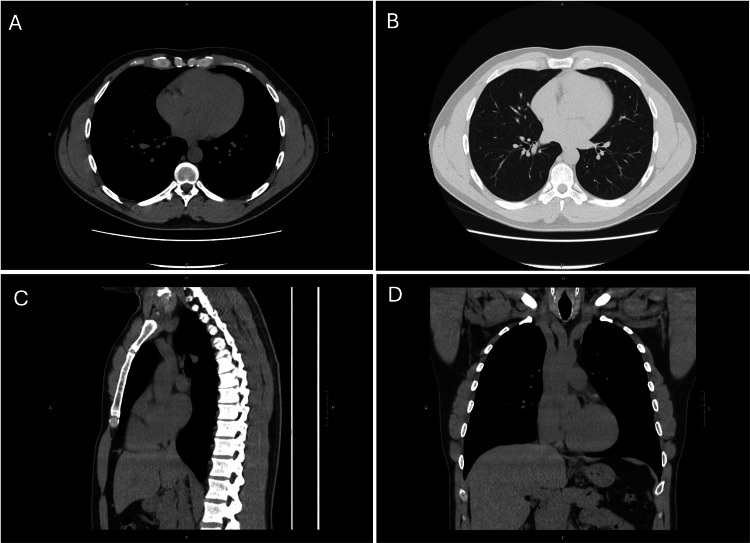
Two-year surveillance CT chest without contrast showing no evidence of intrathoracic metastasis. A: soft tissue body axial, B: lung axial, C: soft tissue body sagittal, D: soft tissue body coronal
